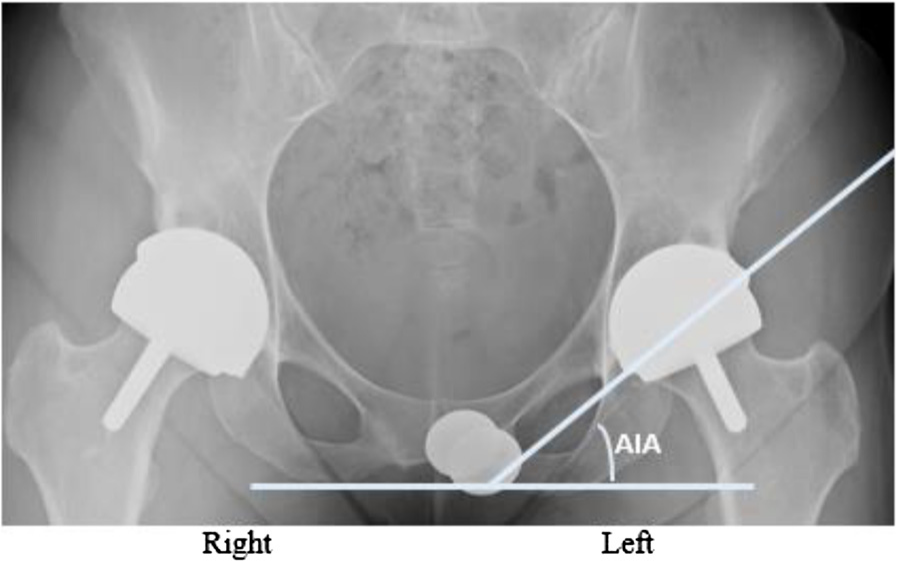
Radiograph Measurement References. Anterior-posterior pelvis radiograph made five years after a hybrid metal-on-metal Corin hip resurfacing on the right hip and two years after a hybrid metal-on-metal Biomet ReCap™ hip resurfacing on the left hip. Better AIA is noted in the most recent HRA on the patients left side
